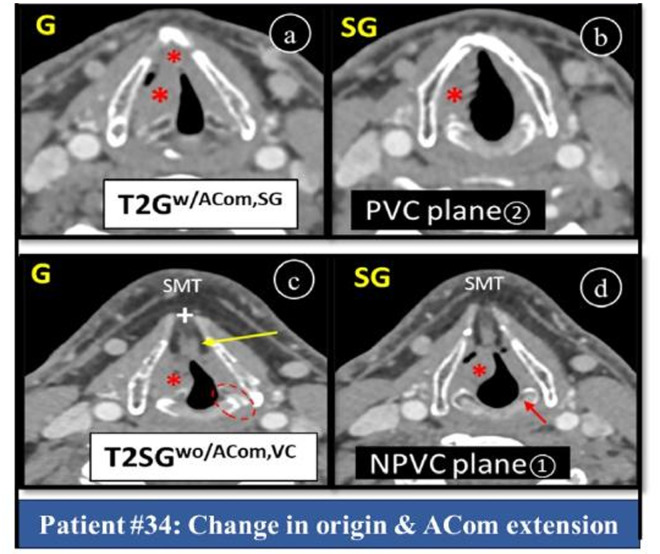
Axial post-contrast CT larynx showing change in origin and ACom involvement due to CT plane. Patient#34: Plane② (a, b) showing ACom invasion, while plane① (c, d) showing normal falsely thickened ACom having fat (long arrow). Asterisks (*) showing areas of tumor extension (G, ACom, SG), dotted oval is CAJ at glottic level, short arrow is SG cricoid cartilage. (+) shows open thyroid notch. Note false anatomical landmarks of G in NPVC plane, i.e., SMT, fat and open thyroid notch. *SpG = supraglottis, G = glottis, w/, w/o ACom=with, without anterior commissure invasion, SG = subglottis, NPVC = non-parallel vocal cord, PVC = parallel vocal cord, SMT = submental triangle, CAJ = cricoarytenoid joint, VC = vocal cord.*
